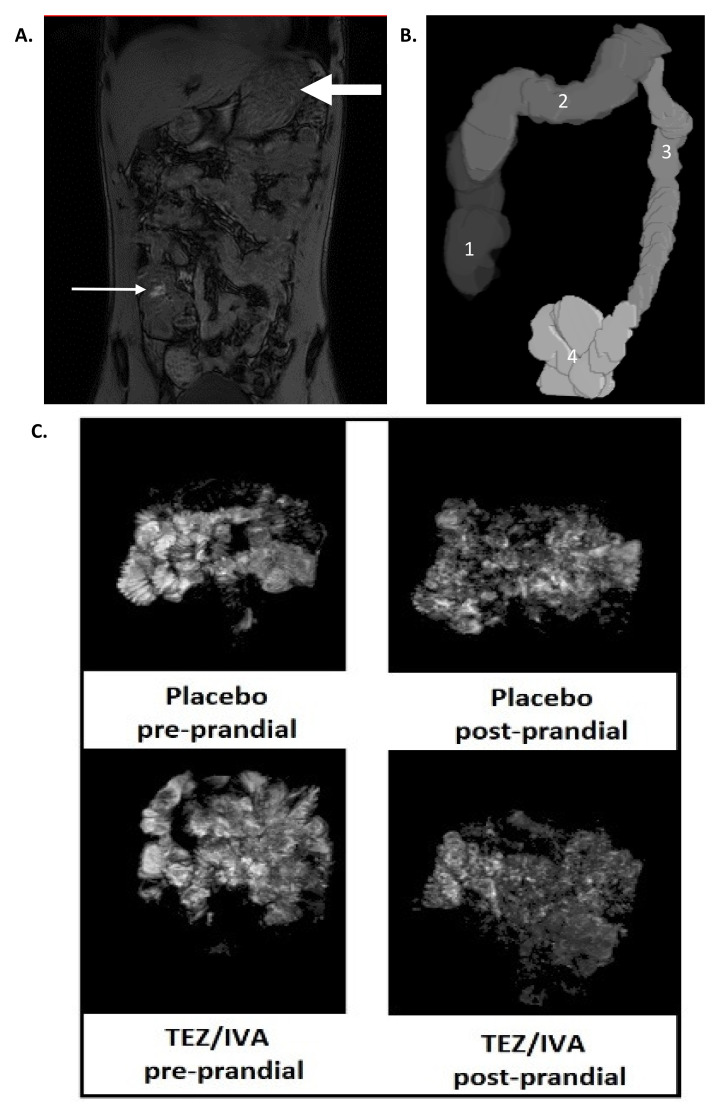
Representative MRI images of (
